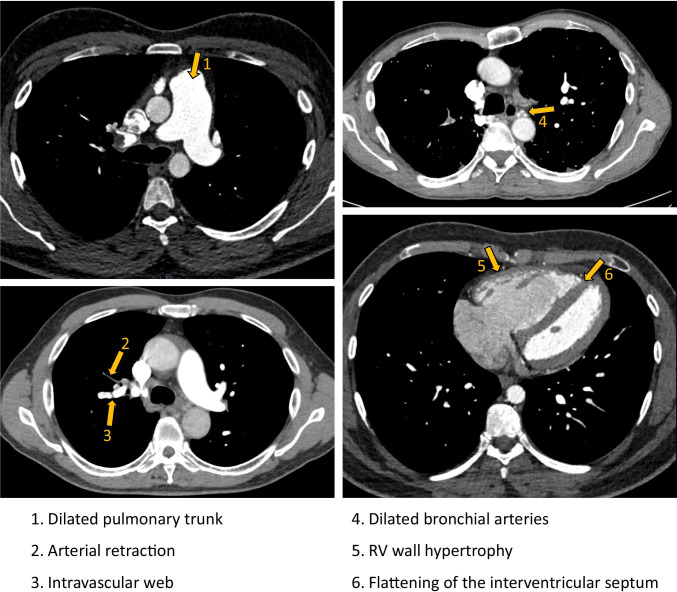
CTPA image showing the six radiological predictors of CTEPH in addition to RV/LV diameter ratio of > 1.0. Abbreviations: CTPA, computed tomography pulmonary angiography; CTEPH, chronic thromboembolic pulmonary hypertension; RV, right ventricle; LV, left ventricle
